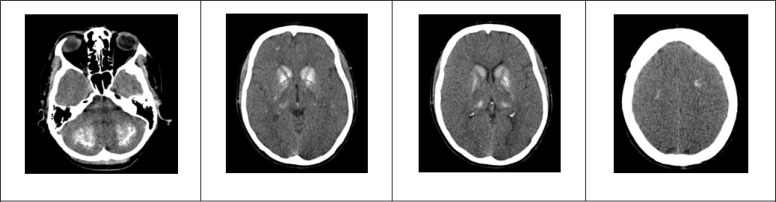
Patient’s axial cuts of brain computed tomography scan without contrast materials.
